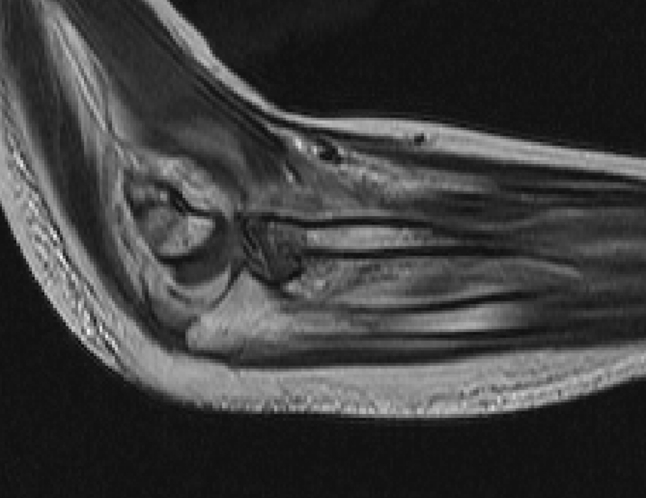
Magnetic resonance image shows the radial head being caught between brachialis tendon and cononoid process
